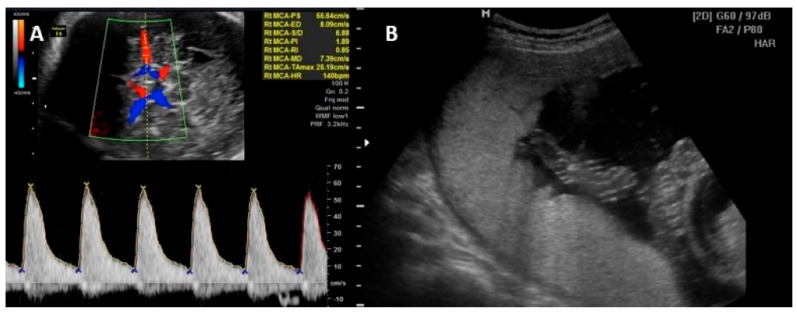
(A) Peak of systolic velocity of the middle cerebral artery Doppler measurement technique. (B) Intrauterine transfusion with the needle in the vein at level of umbilical cord insertion on placenta.
